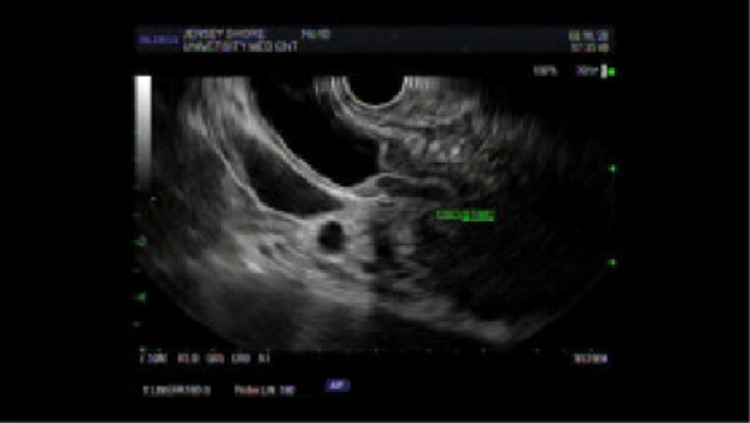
Endoscopic ultrasound of the common bile duct stricture.
